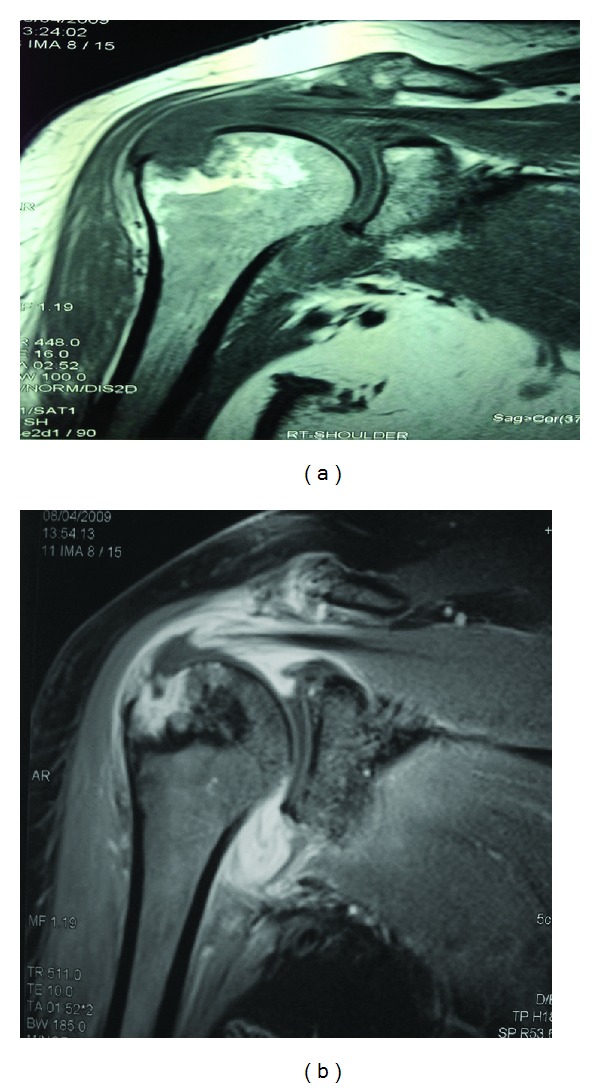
MRI of the right shoulder joint. Coronal T1-weighted (a) and (b) (FS) after IV contrast images of the right shoulder showing dense enhancement of a markedly thickened shoulder joint synovium as well as the subacromial and the subdeltoid bursae with joint effusion is noted. A focal 1 cm area of erosion and underlying trabecular bone enhancement in the greater tuberosity of the humerus and a full thickness tear of supraspinatus tendon with enhancing edges are evident. There is also associated degeneration of the acromioclavicular joint with hypertrophy of its capsule.
